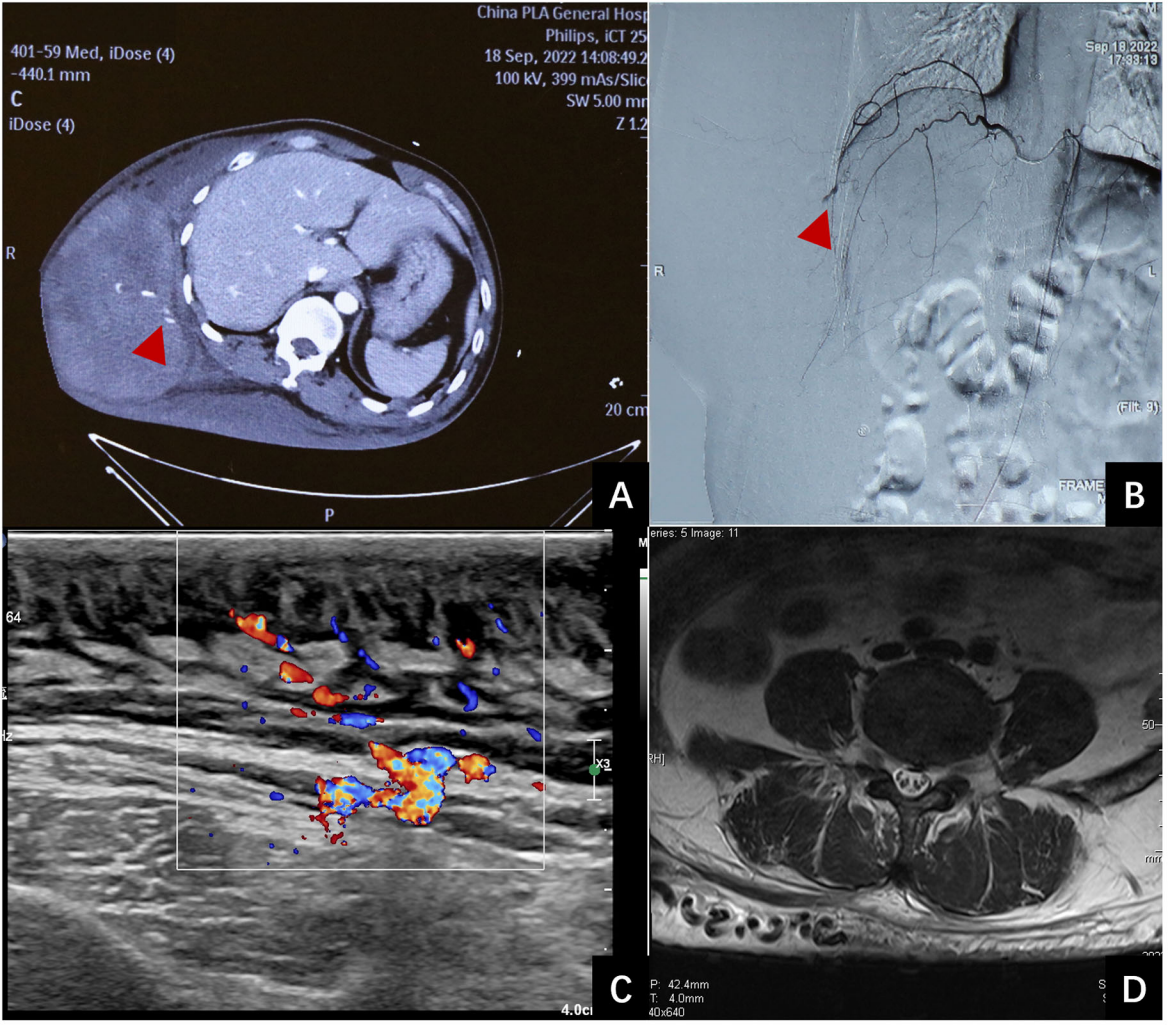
Examination of the patient. (A): Contrast- enhanced computed tomography revealed extravasation of the contrast in the hematoma (red arrow). (B): Emergency interventional radiology showed vascular leakage (red arrows). (C): B-mode ultrasound demonstrates thickening of the skin and subcutaneous fat layer on the back, characterized by uneven echogenicity, with a maximum thickness of 2.7 cm. CDFI reveals abundant blood flow signal within the affected area. Spectral Doppler analysis exhibits arterial- and venous-like flow patterns. An increase in the thickness of the deep fascia is evident, measuring up to 2.9 mm at its thickest point. (D): MRI scan reveals soft tissue thickening and enlarged vascular structures in lower back.
